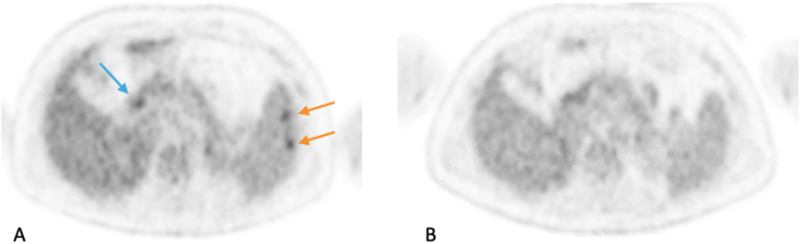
(A) PET-CT showed metabolically active right paracaval lymph nodes (blue arrow) and spleen foci (orange arrows), seventeen days after COVID-19 vaccination. (B) PET-CT showed normalization of previous lymph node and spleen activity, twenty-four days after second COVID-19 vaccination.
